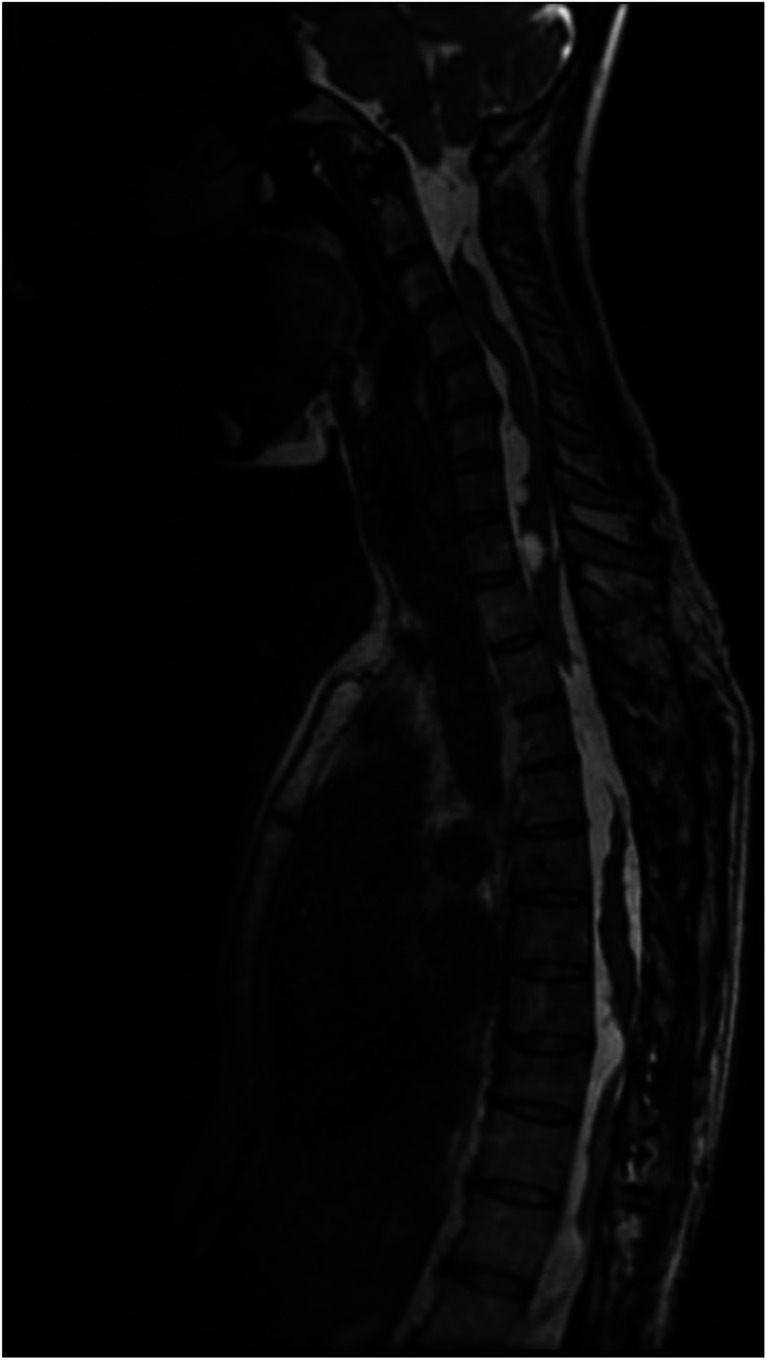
MRI of the whole spine at last follow-up (10 years after initial presentation)
